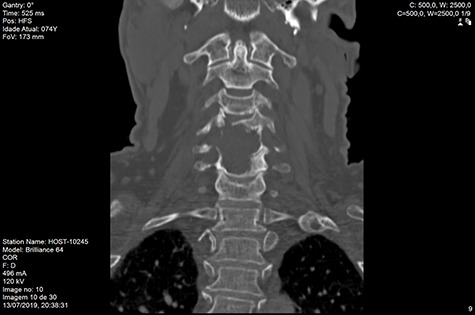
CT coronal D120 post-RT.
